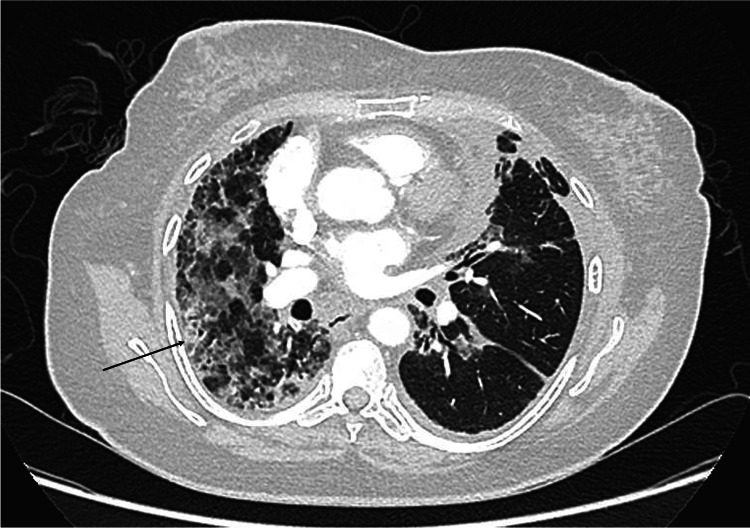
Computed tomography (CT) scan of the thorax done after three cycles of docetaxel, showing patchy areas of ground-glass and consolidation, predominantly in the right lung (indicated by the black arrow)
